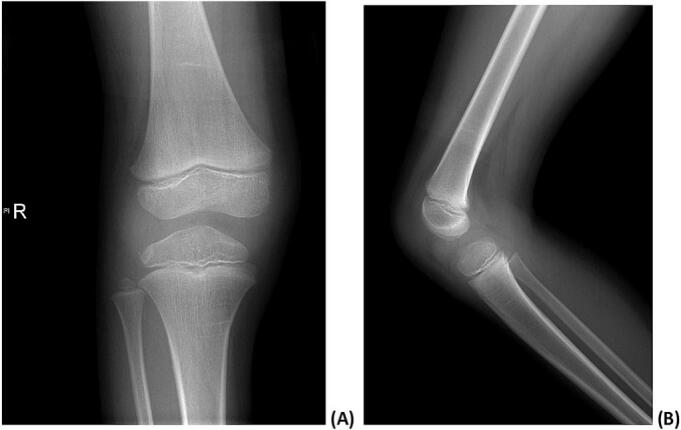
Anteroposterior (A) and lateral (B) radiographs of the right knee that revealed no lesions in the bone or soft tissue.
